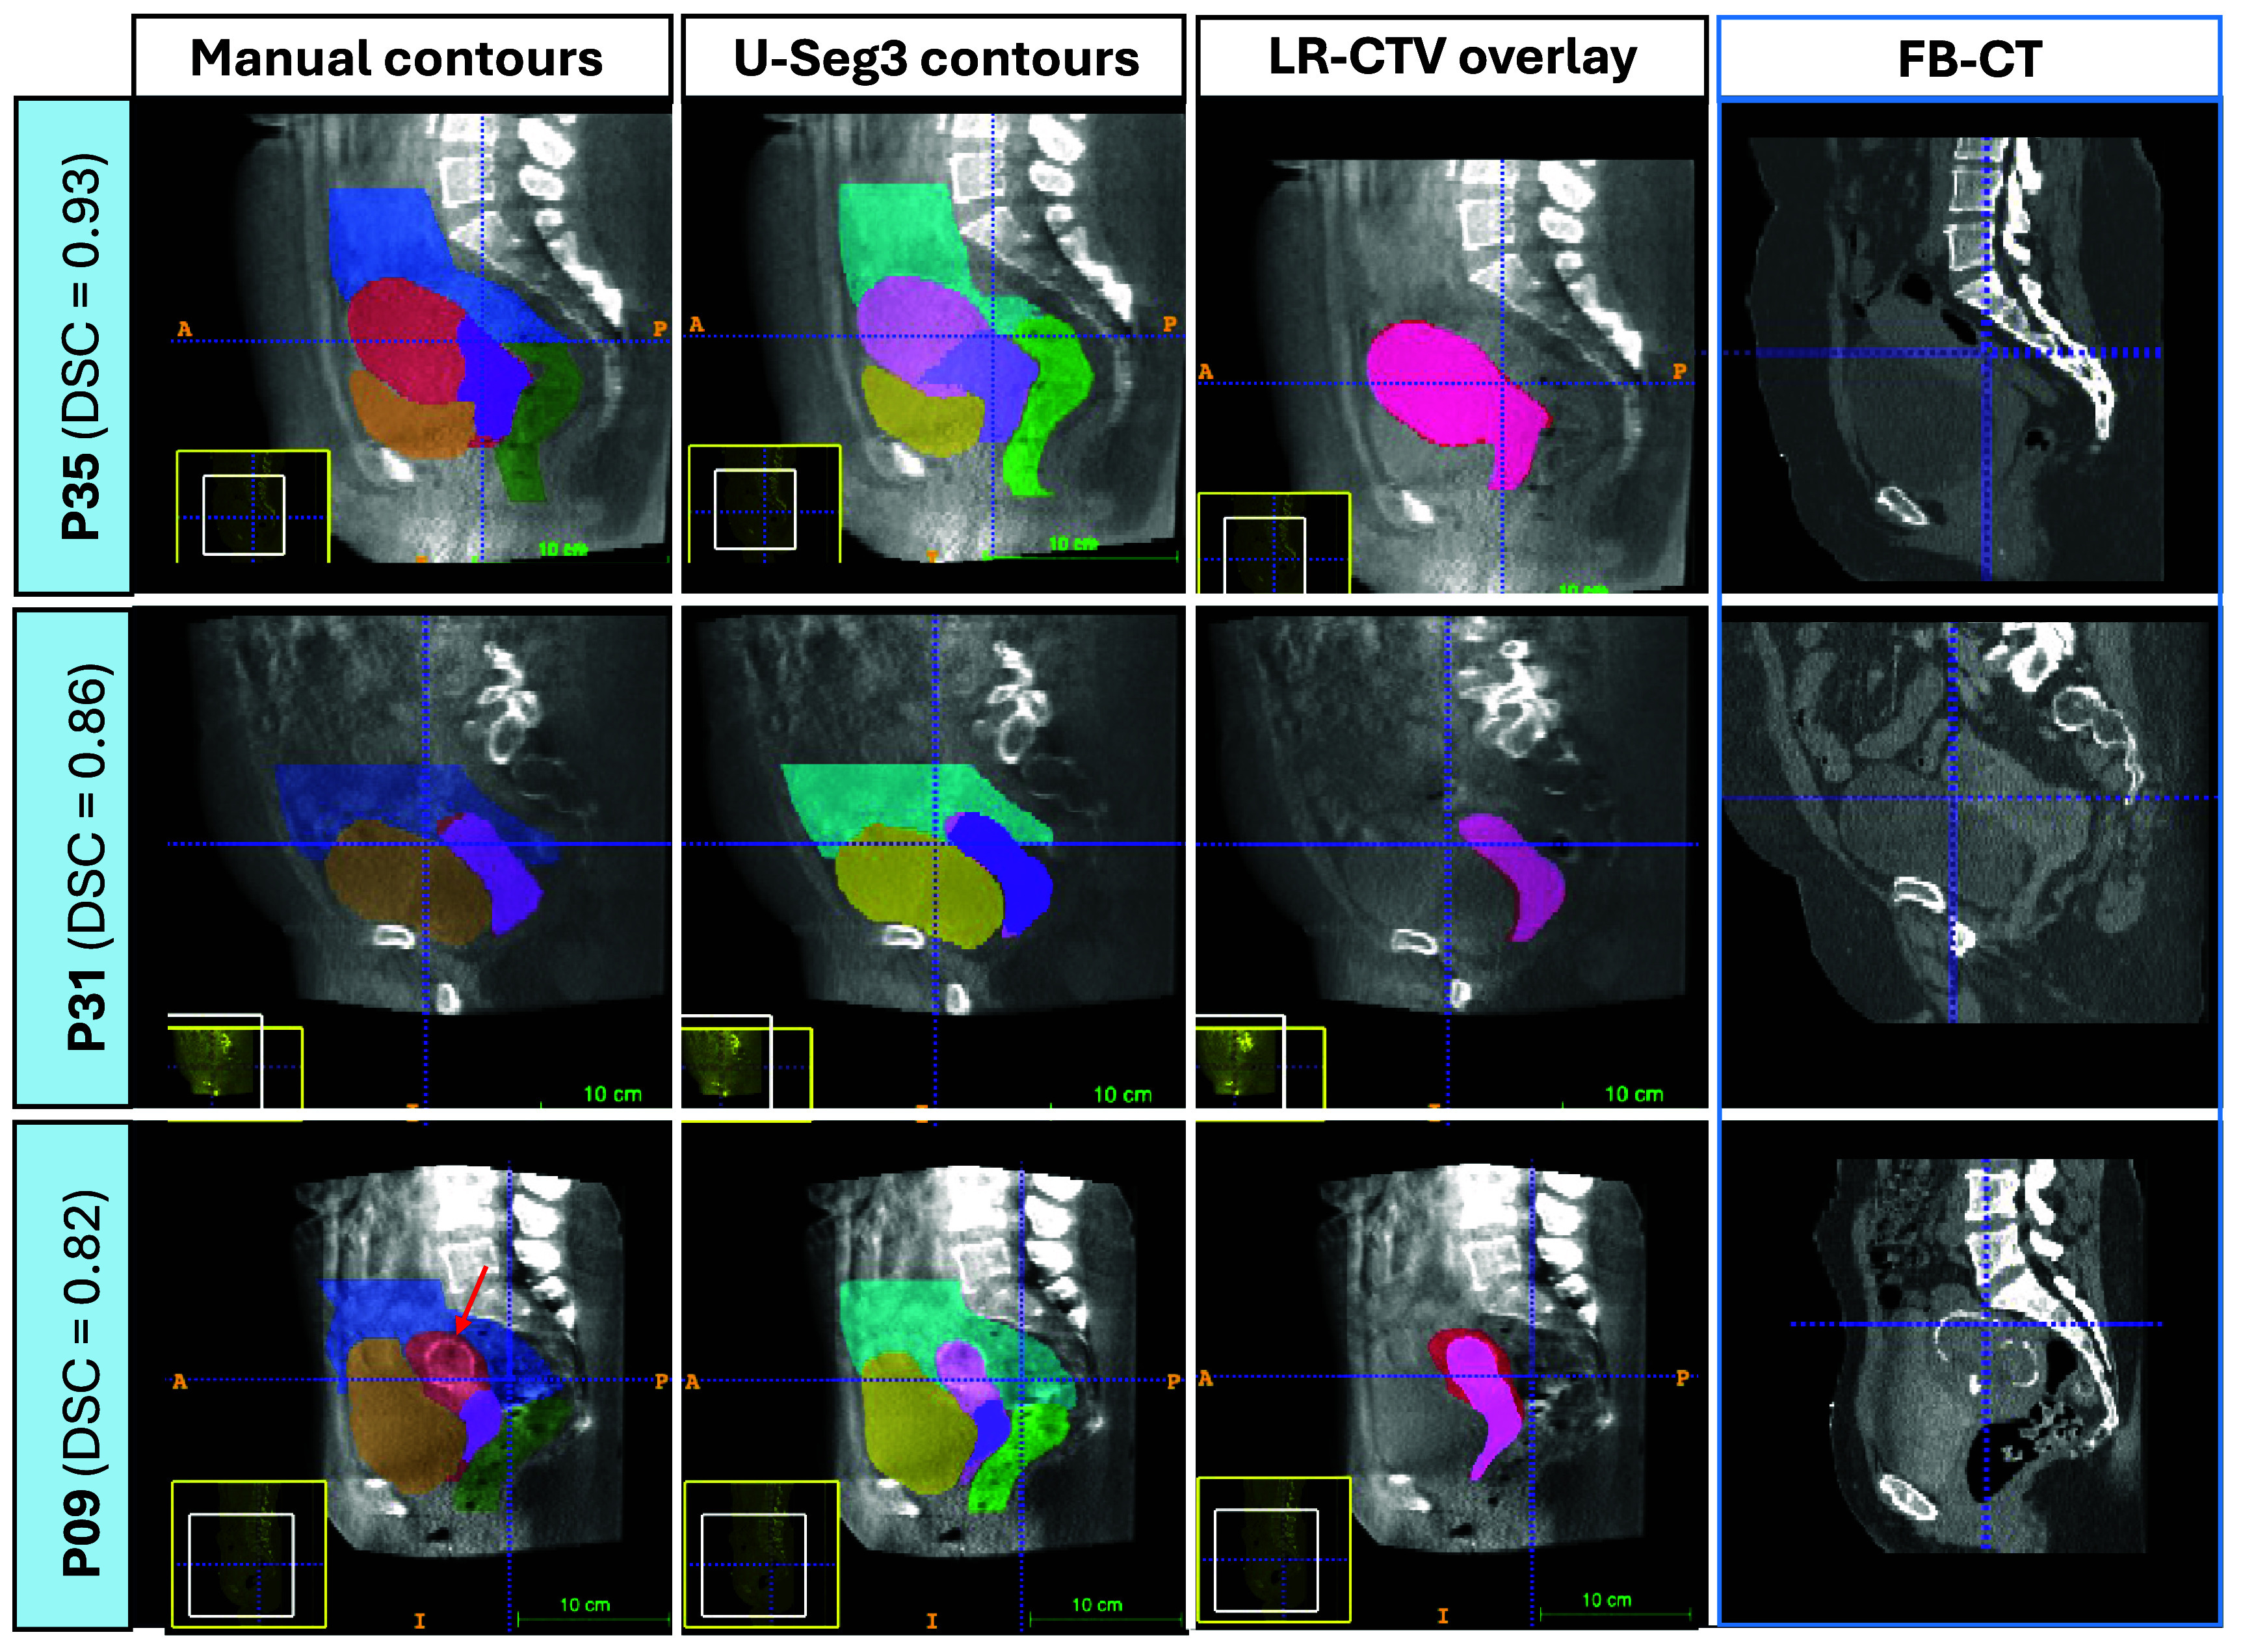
Example U-Seg3 segmentations (col 2) from 3 representative patients. The corresponding manual segmentations are shown in col 1. Col 3 shows the LR-CTV overlay between manual (red) and U-Seg3 (pink) LR-CTV contours. Col 4 shows the corresponding FB-CT that was used as an input to the nnUNet component of U-Seg3. Excellent segmentation performance is demonstrated even when the bladder and LR-CTV volumes are substantially different between treatment and planning (patient 35, row 1), and when the CBCT image quality is degraded by artefacts arising from a hip implant (patient 31, row 2). Patient 9 (row 3) demonstrates an example of U-Seg3 error, where the calcified fibroid (red arrow) was misinterpreted as the uterine boundary. Despite this error, the LR-CTV DSC was still good (0.82) and the optimal plan was still selected given this segmentation as input.
